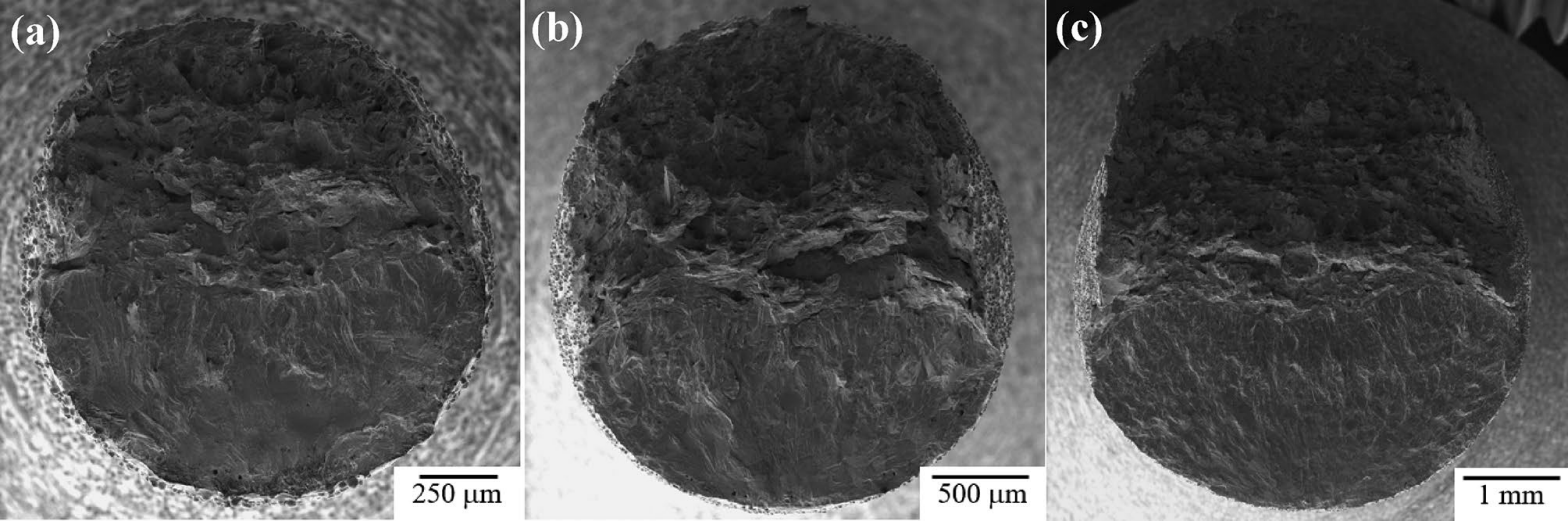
Representative ultrasonic fatigue fracture surface fractography for as-built (a) 1.5 mm CL M2, (b) 2.5 mm CL M2, and (c) 5.0 mm CL M2.
